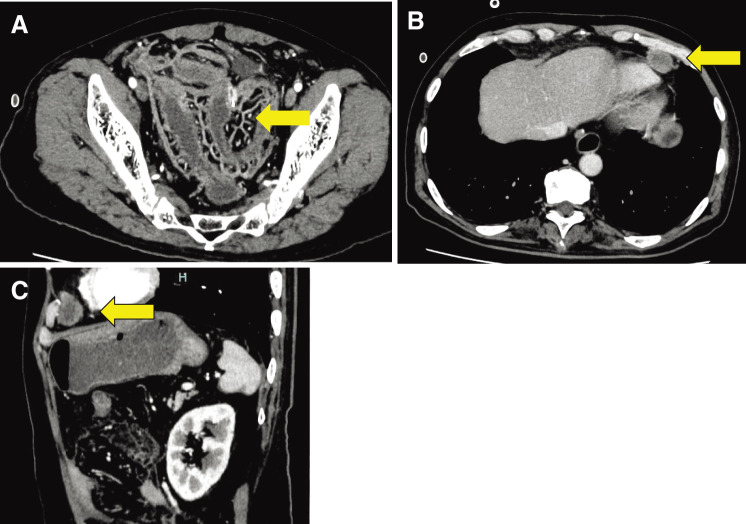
Contrast-enhanced CT (CECT) images (Day 15). The CT study revealed extensive enlargement of pan-mesenteric lymphatic vessels, suggesting rapid lymphovascular invasion by malignant tissue (A, arrow). The mediastinal tumor represented a 200% increase in diameter over 15 days (B and C, arrow).
